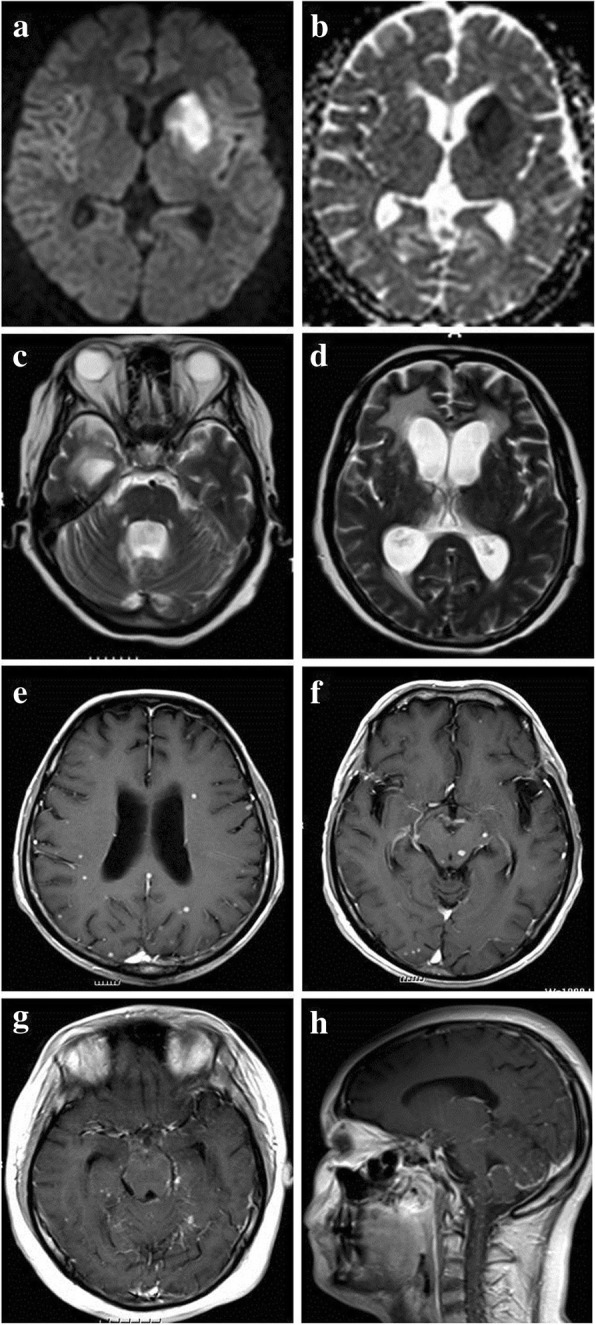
MR presentations of patients with tuberculous meningitis. a-bTBM patient with acute ischemic stroke in basal ganglia (hyperintense in DWI and hypointense in ADC); c-d TBM patient with hydrocephalus; e-f:TBM patient with multiple tuberculomas; g-h TBM patient with basal and cerebellar meningeal enhancement
